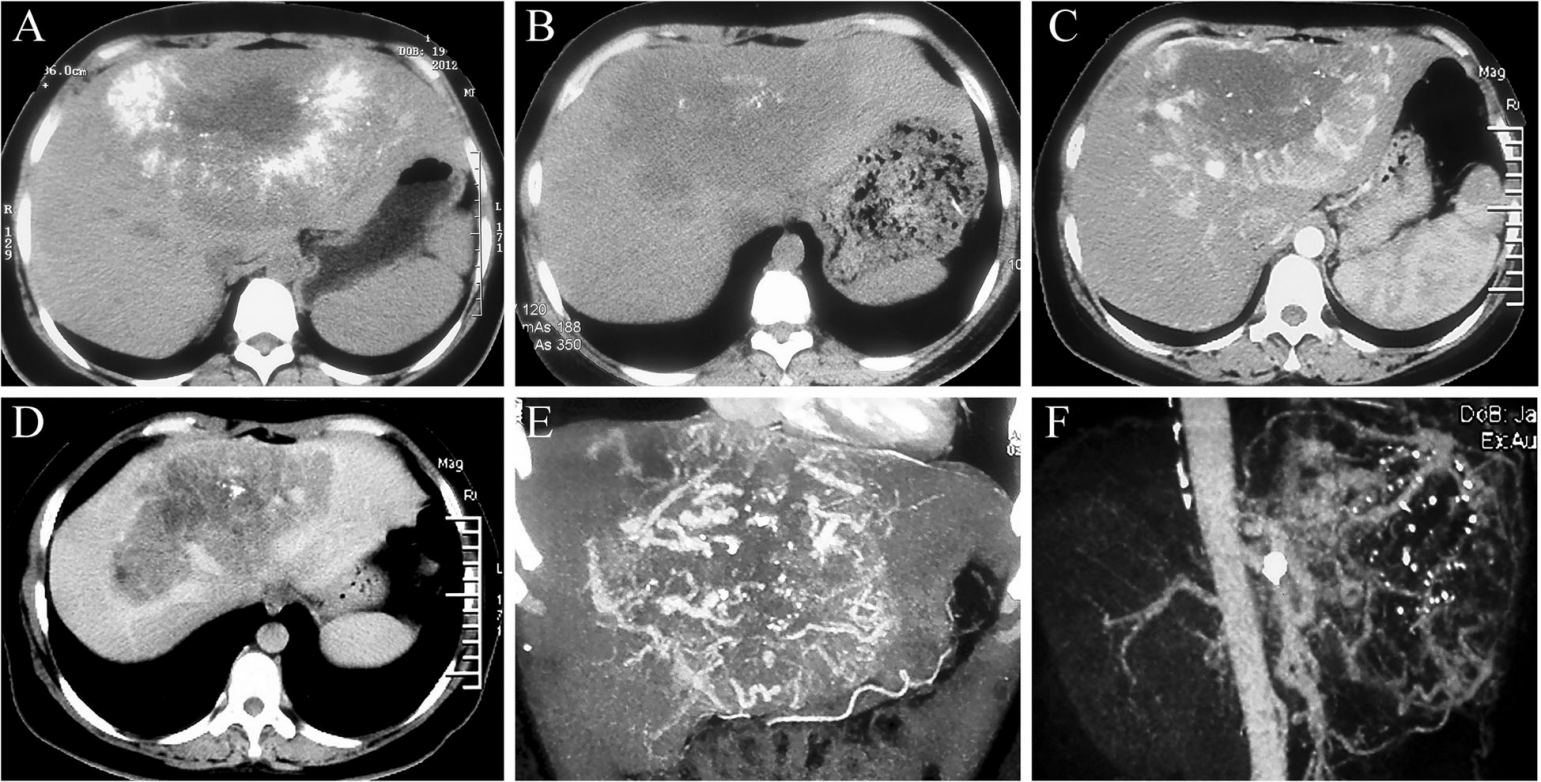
a: post-TACE CT scan revealed densely deposited lipiodol inside the tumor; b: CT scan revealed a huge solid hypodense mass in the liver; c: The lesion was hypodense during the portal phase; d: The lesion showed a strong heterogeneous enhancement in the arterial phase; e, f: Angiography demonstrated a round hypervascular mass with many feeding arteries
